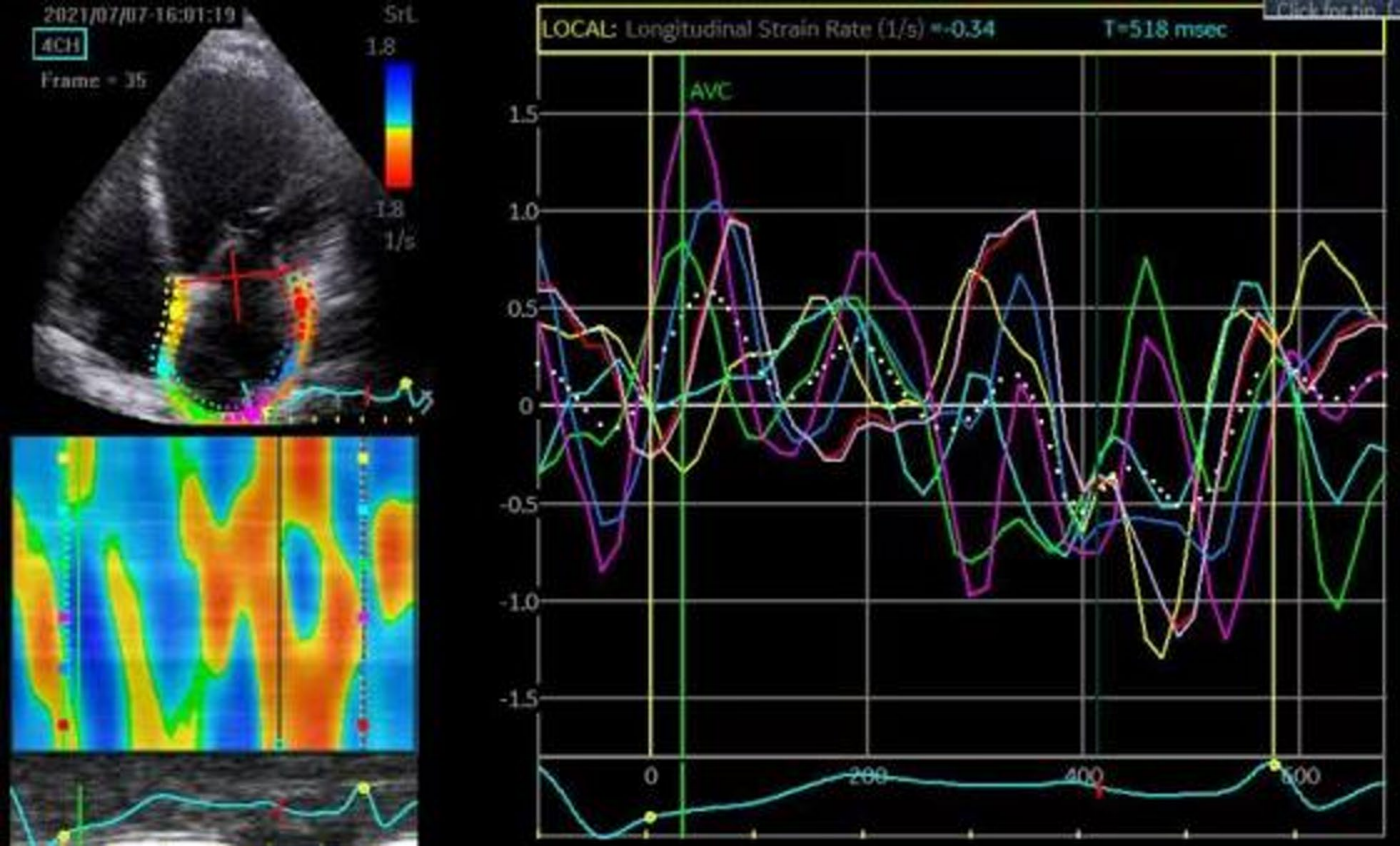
Measurement of LA strain and strain rate in apical four chamber view
